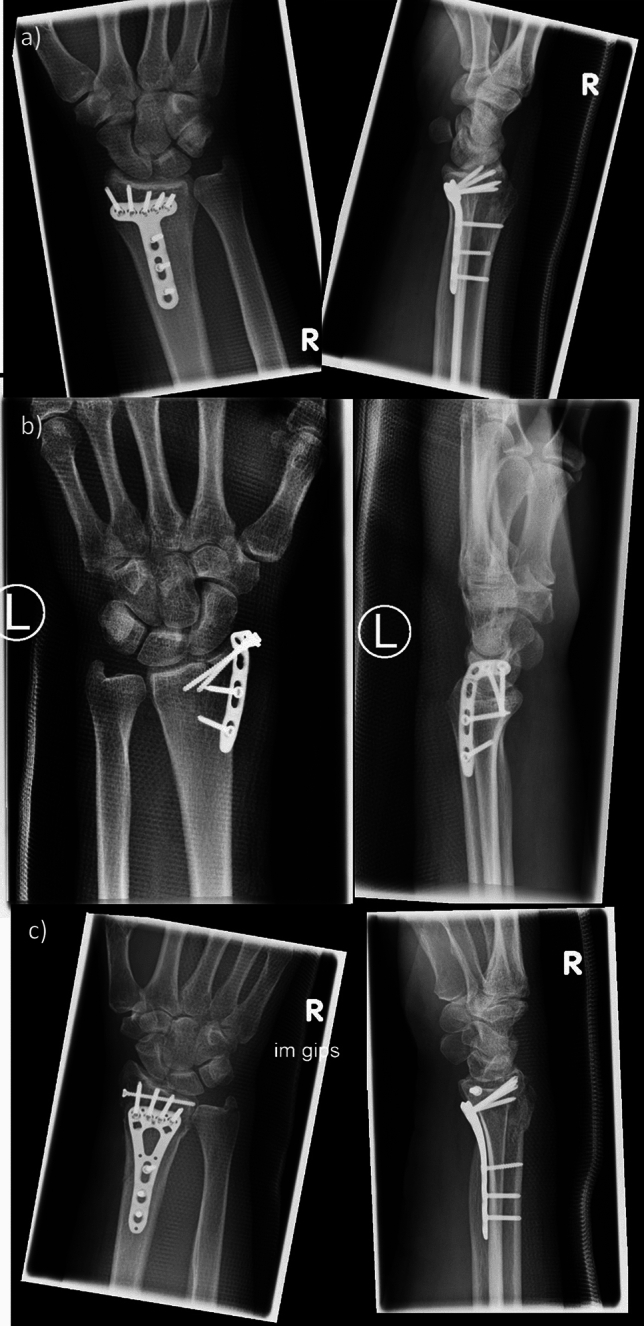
Postoperative surgery X-ray images. As there nearly exist no ct scans postoperatively, only X-ray images were shown and used for the assignment test. a Distal radius fracture type a, b fracture AO b, and c fracture type c
